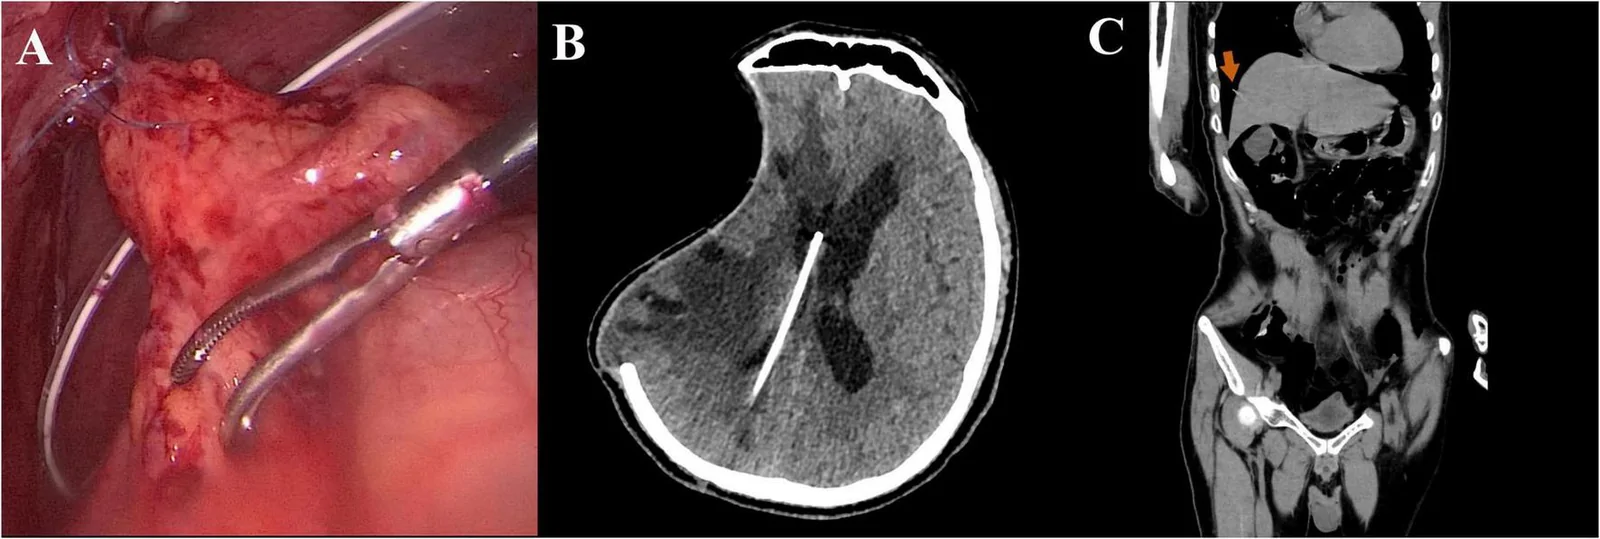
(A) Final position of the peritoneal catheter after revision. (B) Postoperative axial cranial CT. (C) Postoperative coronal abdominal CT.
